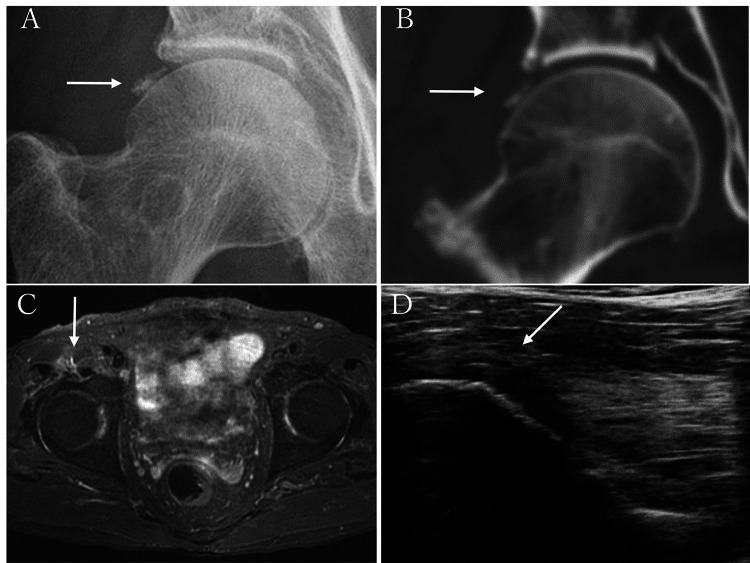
Radiographs, CT, MRI, and an ultrasound examination of the hip. Anteroposterior radiograph of the right hip showing calcification near the superior lip of the acetabulum (arrow, A). Computed tomography of the right hip showing calcification in the reflected head of the rectus femoris (arrow, B). Magnetic resonance imaging coronal T2-weighted fat-suppressed image. Magnetic resonance imaging showed a signal change in the reflected head of the rectus femoris with an inflammatory change of the peripheral soft tissue (arrow, C). Ultrasound longitudinal static scanning showed a calcification with an inflammatory change of the peripheral soft tissue (arrow, D).
